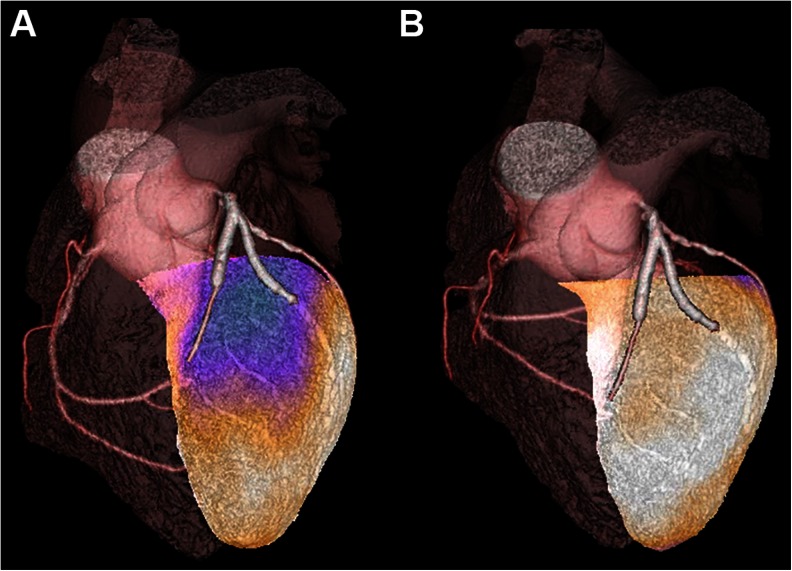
Example of hybrid SPECT/CTA under adenosine pharmacological stress in a 58-year male patient with two coronary stents (one implanted in the proximal left anterior descending [LAD] and one in the first diagonal branch) (a) A myocardial perfusion imaging was requested for investigating the start of angor similar as described before stent implantation. The CTCA did not show any intra-stent stenosis, but failed to visualize a small branch from the LAD which was recovered by the stent mesh and which was not visible anymore on CTCA, responsible for the symptomatic ischemia with a stress-induced perfusion defect (summed stress score = 10; summed rest score = 4; summed difference score = 6). There was unfortunately no possibility to revascularize this small branch and, consequently, maximal risk factor reduction and physical activity training were recommended. (b) After 9 months, myocardial perfusion imaging was repeated and fused with the same CTCA and regained normal perfusion at stress and rest, without ischemia and scar
